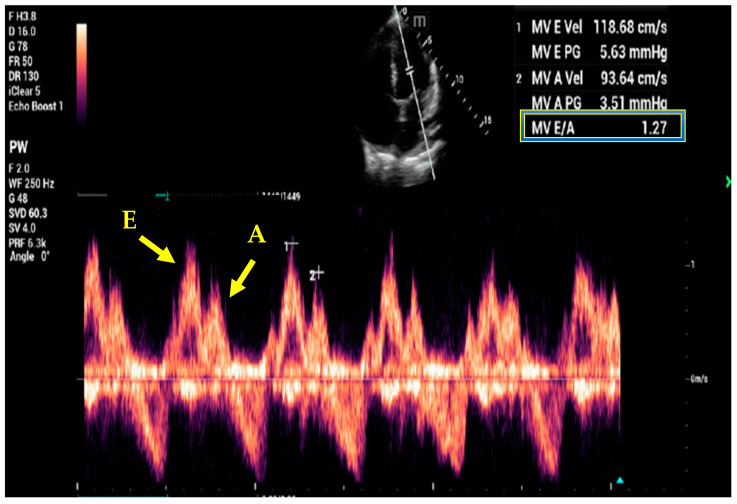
Doppler analysis showing mitral valve inflow including E (passive filling during early diastole) and A (active filling during late diastole as the LA contracts) waves with a normal E/A ratio.
